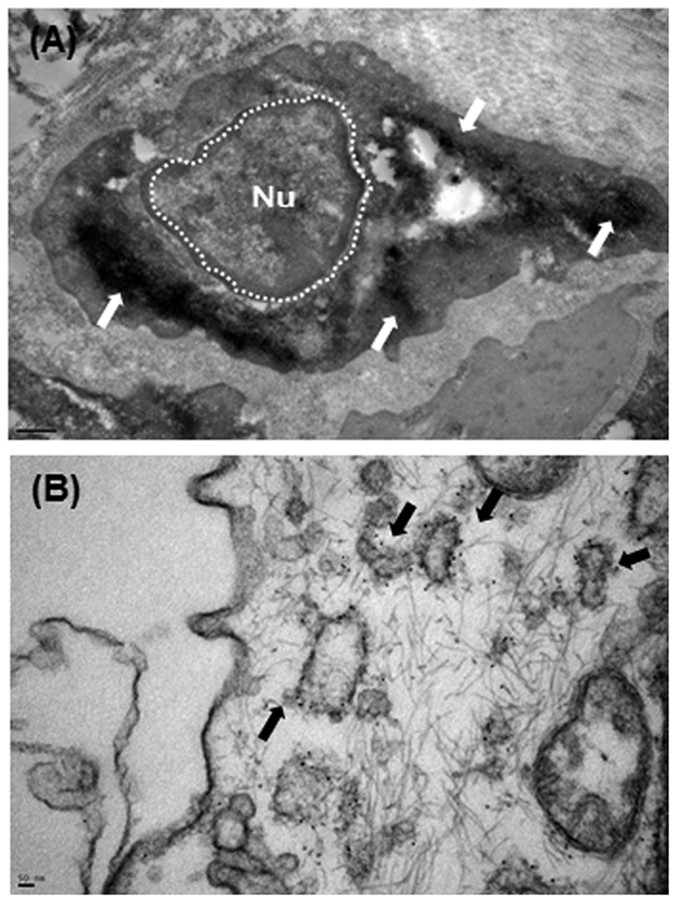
Representative TEM images of (A) immunoperoxidase/diaminobenzidine methods and (B) immunogold enhancement showing ultrastructural localization of PSMA. Bar in (A) 1 μm. Bar in (B) 10 nm.
